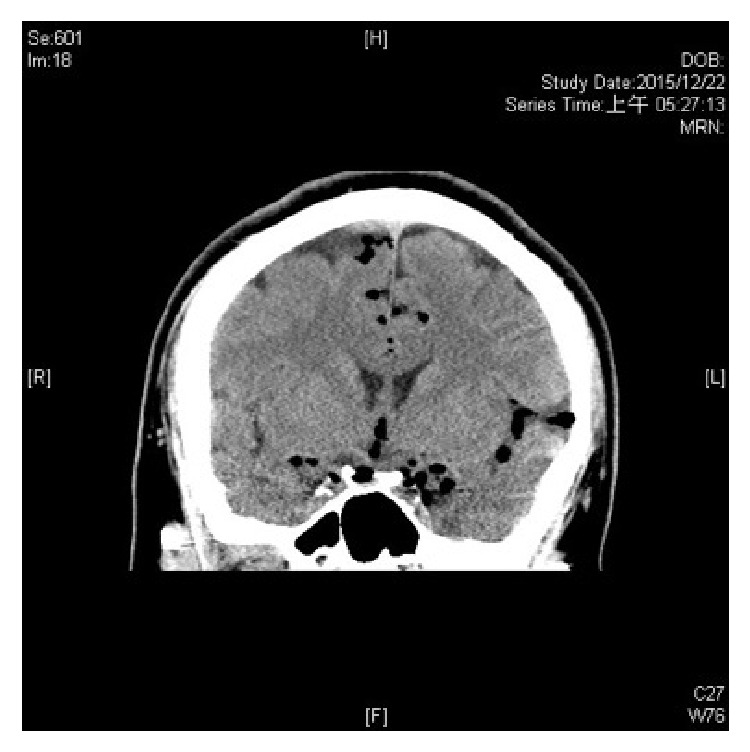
The transverse view of the CT scan shows air accumulation in the subarachnoid space indicating a pneumocephalus.
